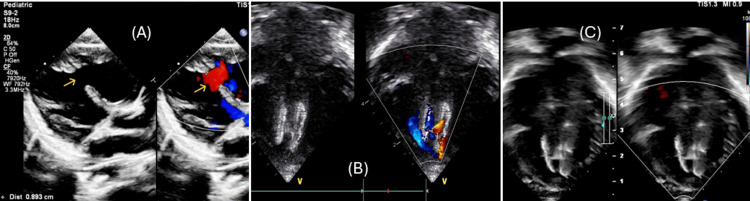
Case 1 echocardiogram images (A) ventricular septal defect (VSD) assessment on echocardiogram on the parasternal long axis view. (B) Device immediately after deployment. (C) Device after six months of deployment on the apical four-chamber view. The yellow arrow shows the VSD.
